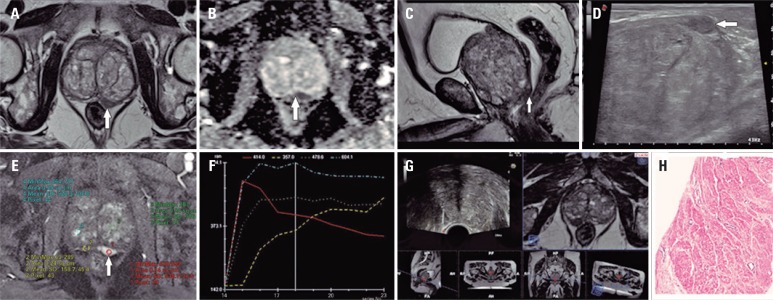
A 73-year-old man with prostate specific antigen level of 6.6ng/mL, palpable nodule on digital rectal examination and negative prior biopsy. Multiparametric magnetic resonance imaging of the prostate shows a well-defined nodule, with very low signal intensity in the peripheral zone on T2-WI (A and C), intense restricted diffusion demonstrated by a low signal on apparent diffusion coefficient map (B) and hypervascularized pattern, with an early wash-in followed by wash-out curve (E and F). Real time imaging fusion ultrasound/magnetic resonance imaging technique: the nodule seen as a low-signal lesion in the peripheral zone of the prostate on T2-WI (C) appears as a round hypoechoic nodule on ultrasound (D). Systematic biopsy with additional samples of the suspicious area was performed (note that magnetic resonance imaging images were inverted during the procedure) (G), and pathological results demonstrated a pure prostatic leiomyoma (H)
